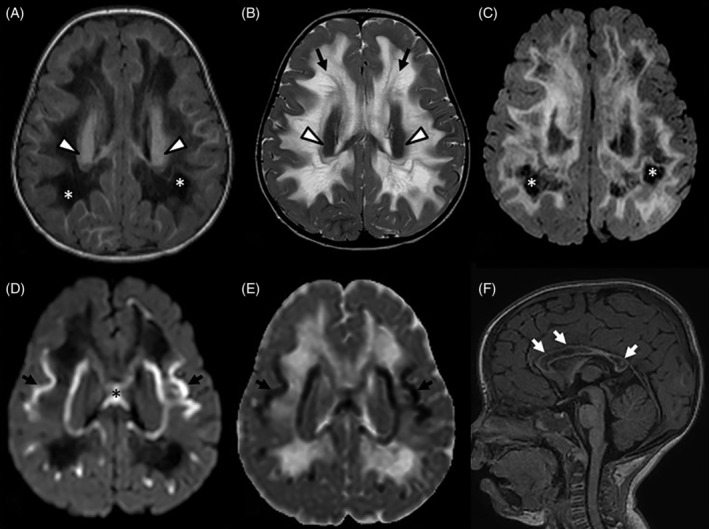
Axial spin‐echo T1‐ (A) and Turbo Spin‐Echo T2‐weighted sequences (B) show bilateral and confluent involvement of deep and peripheral supratentorial white matter. Note bilateral vacuolating pattern with cerebrospinal fluid‐like signal intensity on T1‐weighted and FLAIR sequences (asterisks in A and C) and the tigroid‐like pattern of the frontal deep white matter on T2‐weighted sequence (black arrows in B). The deep white matter of the corona radiata is spared bilaterally (white arrowheads in A and B). Diffusion weighted imaging (DWI; *b* = 1000) and apparent diffusion coefficient map show bilateral rims of restricted signal in the deep and juxtacortical white mater (black arrows in D and E) involving the trunk of the corpus callosum (black asterisk in D). The corpus callosum is diffusely vacuolated, with partial sparing of part of the isthmus (white arrows in F)
